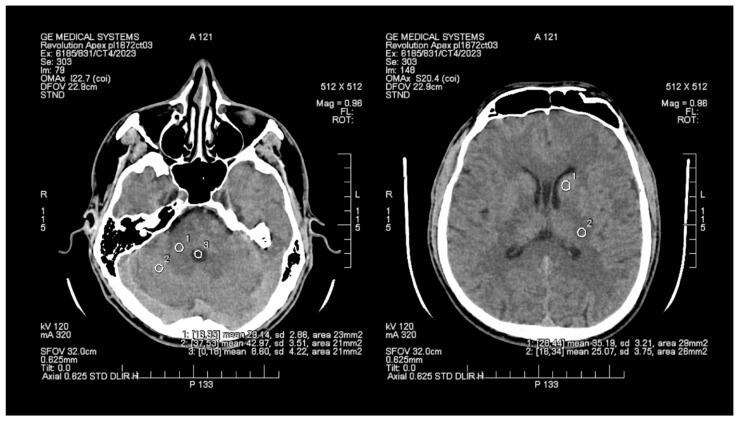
Images of head CT with marked ROIs located in BGA (basal ganglia area) and PCF (posterior cranial fossa) obtained using DLIR.
